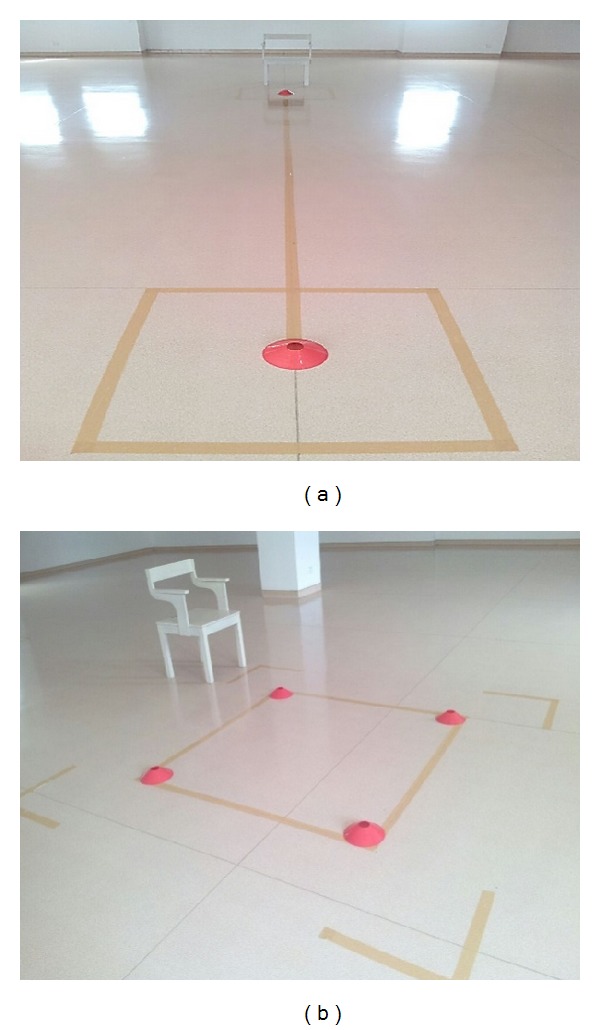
(a) and (b) show the tracks for ST and CT, respectively.
